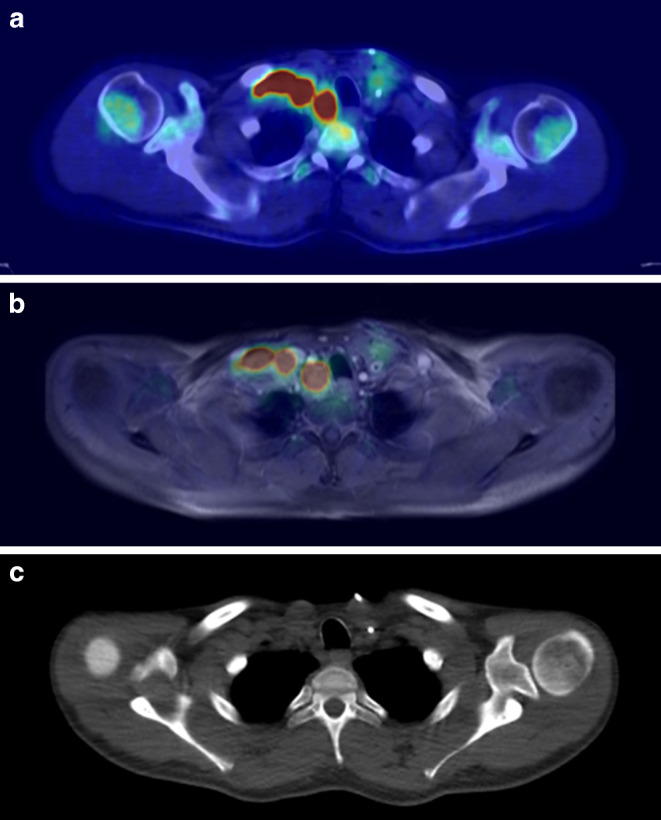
Imaging of patient 4. a FDG-PET/CT before chemotherapy. b FDG-PET/MRI before chemotherapy. c Planning CT after chemotherapy
